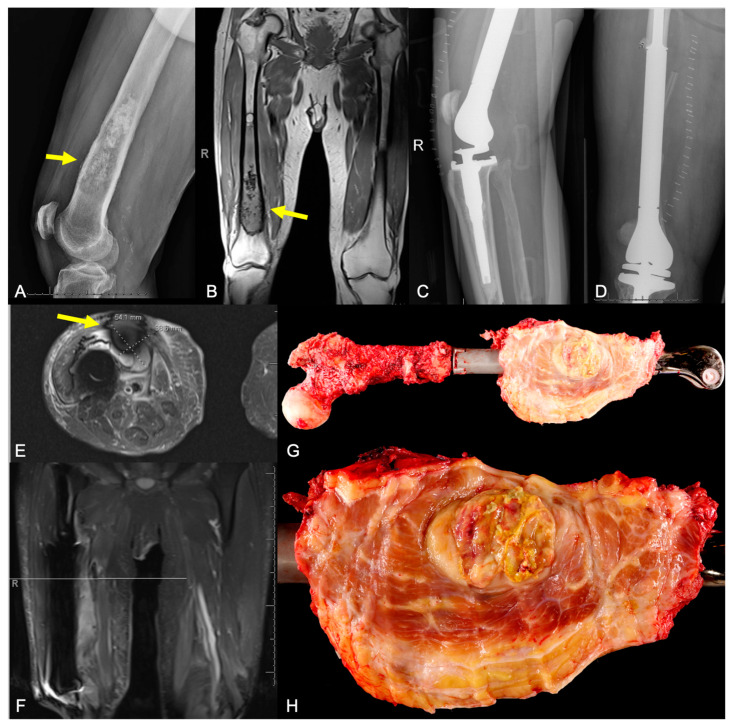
Imaging of a 77-year-old male with history of dedifferentiated chondrosarcoma of the femur treated with wide resection and endoprosthetic reconstruction, now presenting 6 months post-operatively after feeling a soft tissue mass in the region of the original tumor. Initial (A) radiograph and (B) MR image of the tumor. (C,D) Post-operative radiographs after tumor resection and endoprosthetic reconstruction. (E,F) MR images confirming tumor recurrence (type 5A failure), necessitating revision surgery, prosthesis removal, and total femoral replacement. (G,H) Gross pathology images of the removed tumor demonstrate encasement of the prosthesis.
